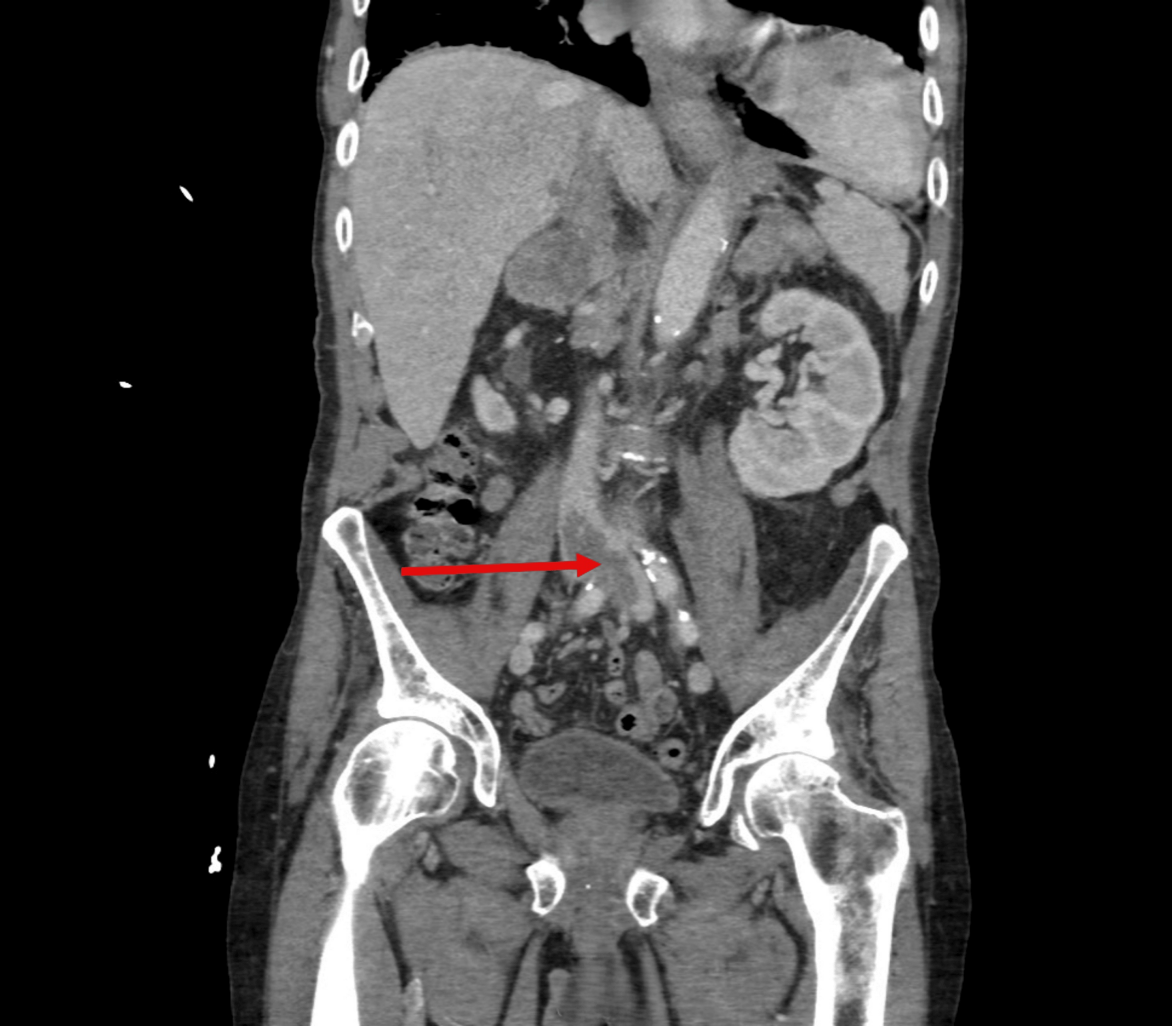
Coronal contrast CT abdomen showing a large IVC thrombus (arrow). IVC: inferior vena cava
